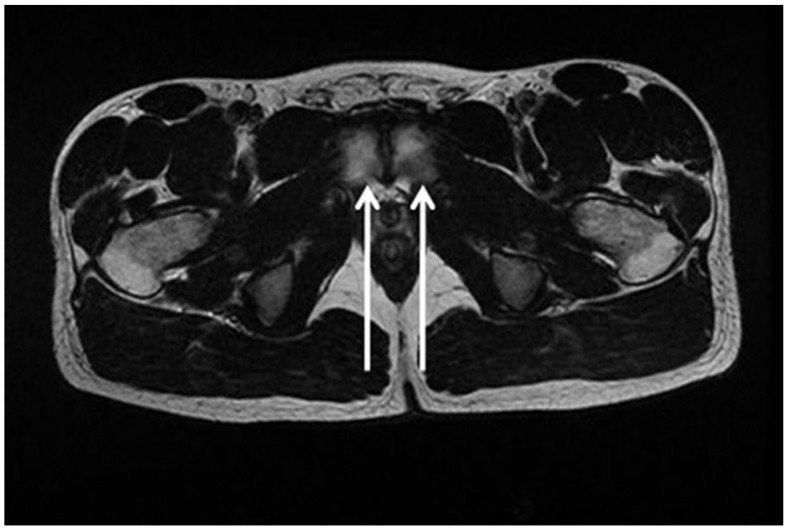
Axial oblique T2 MRI showing bone marrow oedema extended across the surface, in the antero-posterior direction of both the pubic branches (arrows).
